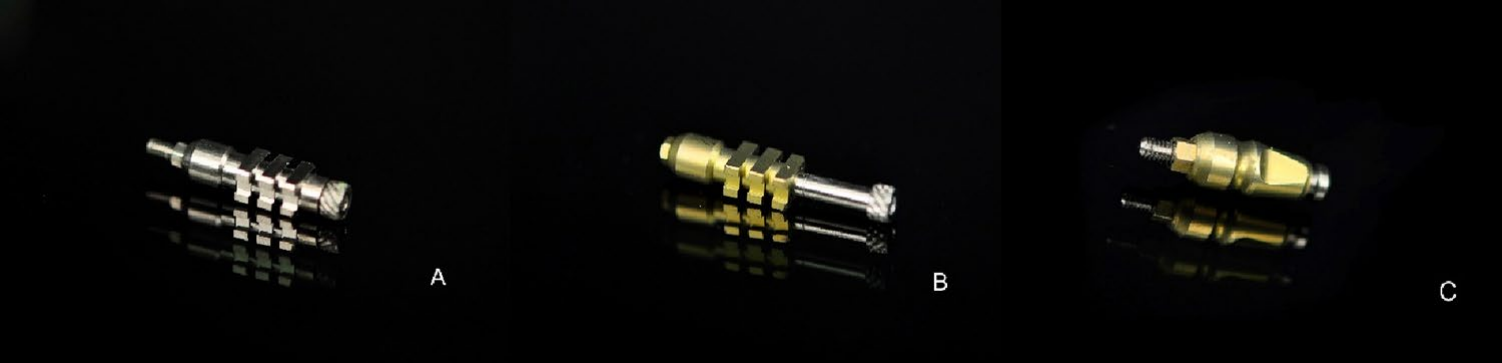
The three transfers under analysis: TOT A, COT B and CT C, respectively
